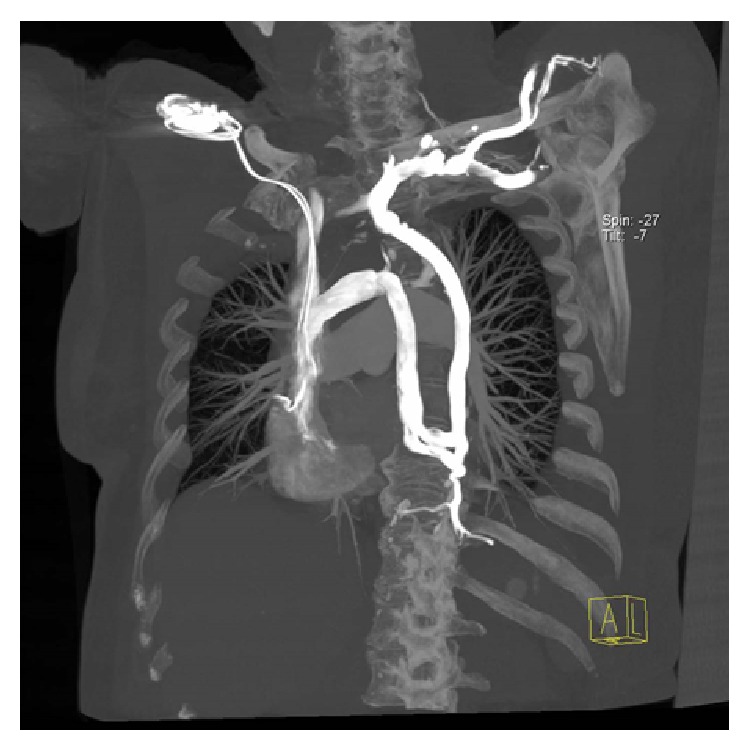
Reformatted CT angiography image shows the anomaly in the venous phase.
